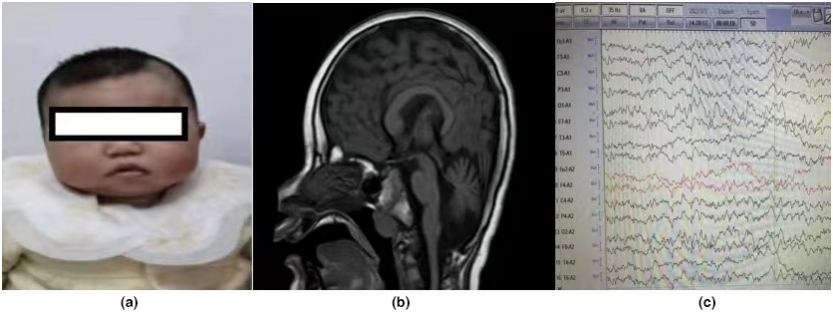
(a–c) The representive clinical characteristics of patient2: (a) Microcephaly with wide‐set eyes, wide nasal bridge, small nose andhigh‐arched palate. (b) Decreased volume of the bilateral cerebellarhemispheres and brain stem decreased on brain MRI. (c) EEG unremarkable.
